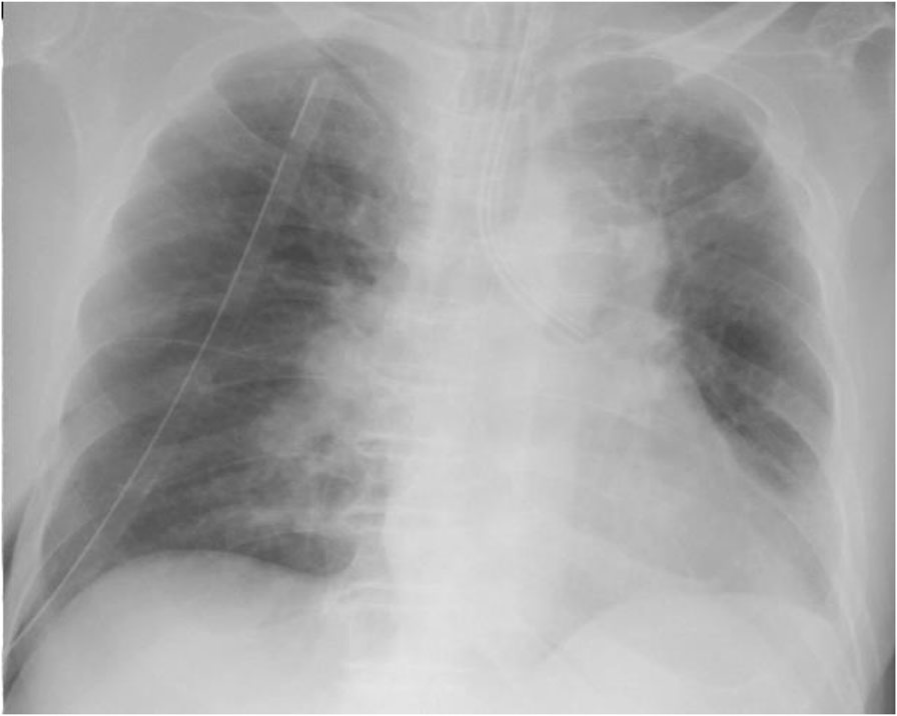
Chest radiograph after the wedge resection of the lung showing excellent lung expansion
